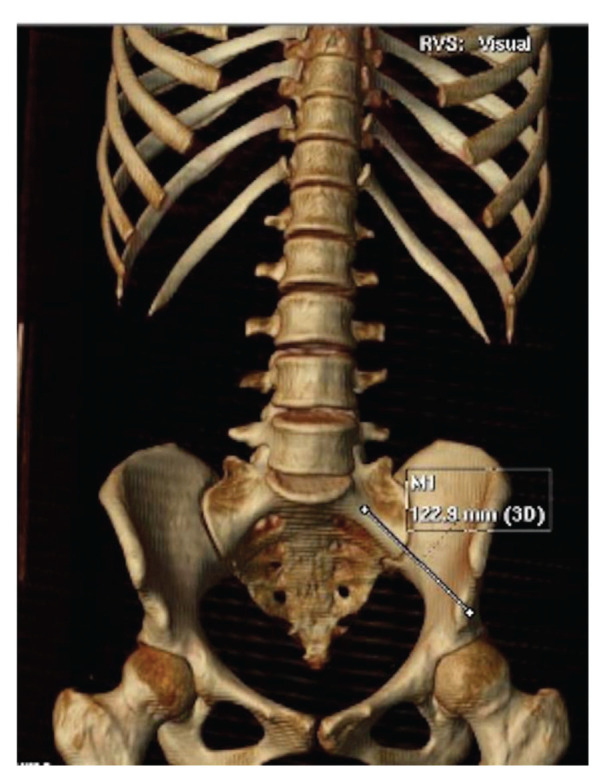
Example of S2AI screw length on CT 3D (shown by white line).
